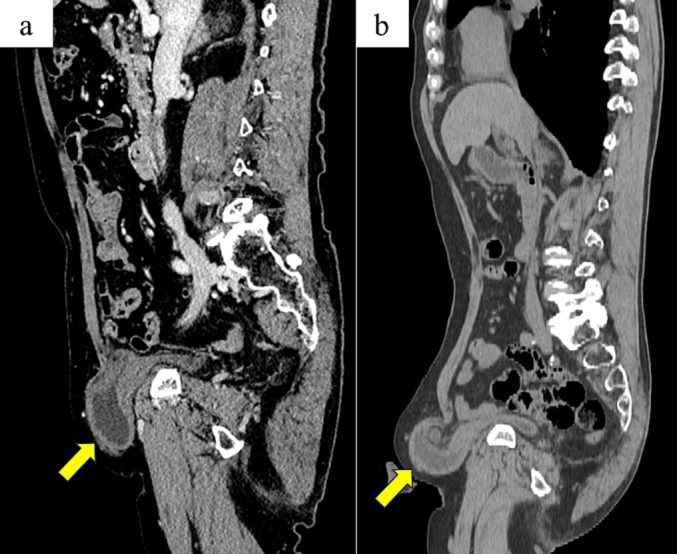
Images on computed tomography (sagittal view). a. Asymptomatic right inguinal bladder hernia (arrow). b. Right inguinal bladder hernia exacerbated (arrow) after pancreatic surgery.
